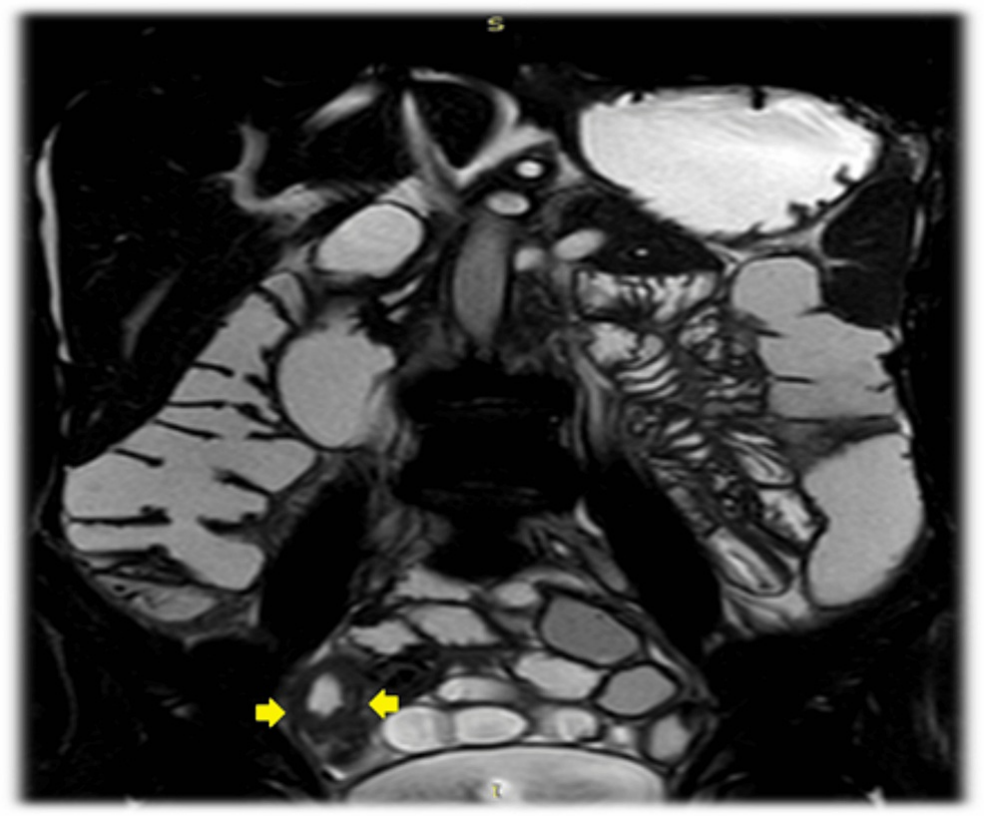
Small bowel enteroclysis magnetic resonance imaging (MRI) A coronal selected image of MR enteroclysis showing ileal wall thickening (yellow arrows) in the right lower abdominal quadrant
